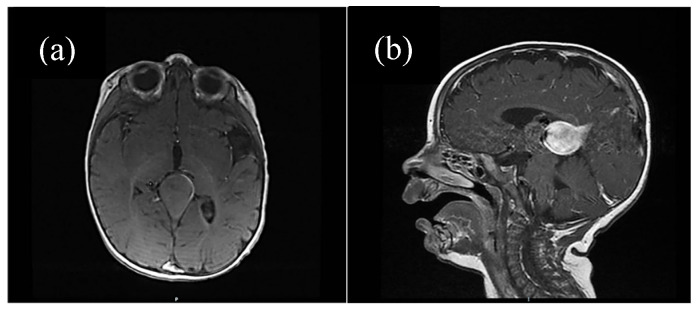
(a) Native cerebral MRI (transverse section), (b) contrast cerebral IRM (sagittal section). Vascular aneurysmal dilation at the level of the quadrigeminal tank, with no significant compressive effect, no thrombosis, no infra/supratentorial lesions. Asymmetrical ventricular system but normally positioned midline structures. Ependymal cyst located in the atrium of the LV. Asymmetry of the temporal lobes due to incomplete gyration.
